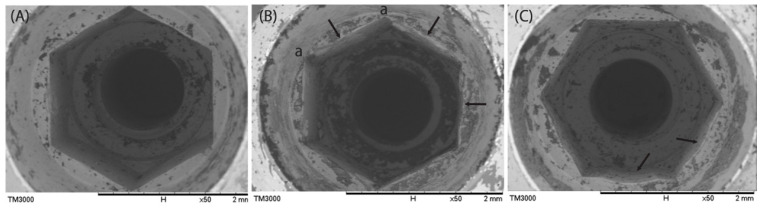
SEM images of implants after removal of fractured screws via three different methods: (A) Ultrasonic scaler, (B) fissure bur, and (C) screw removal kit. Arrows point to damaged sides and letter “a” indicates the jeopardized angle of the hex.
